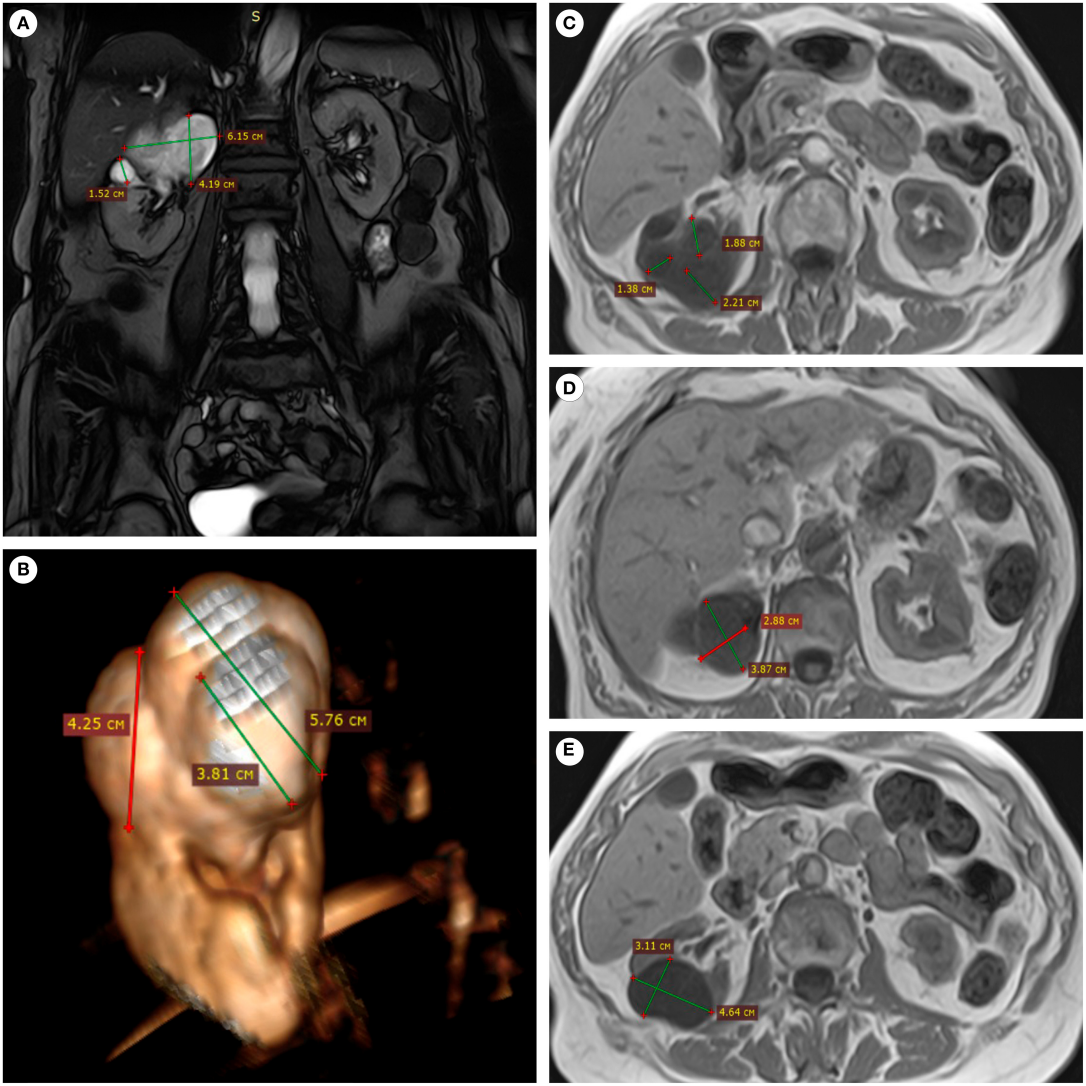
MRI of the patient's abdominal cavity (without contrast). (A) In the upper pole of the right kidney, the lesion of an irregular shape and inhomogeneous structure was connected with the pelvicalyceal system, coronal projection, SSFP mode. (B) The lesion had an irregular shape and a bumpy surface, 3D-reconstruction from the SSFP series. (C–E) The multinodular nature of the lesion and clear boundaries were determined in axial sections, T1 mode.
